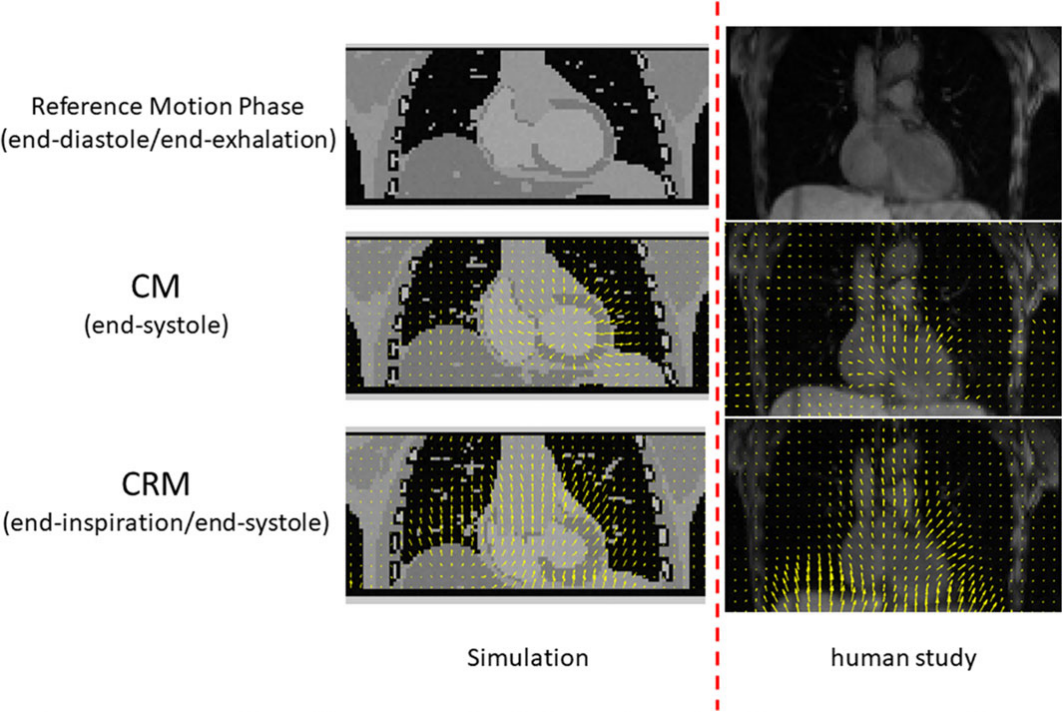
Coronal MR images for two different motion phases along with the estimated motion fields between the two motion phases
